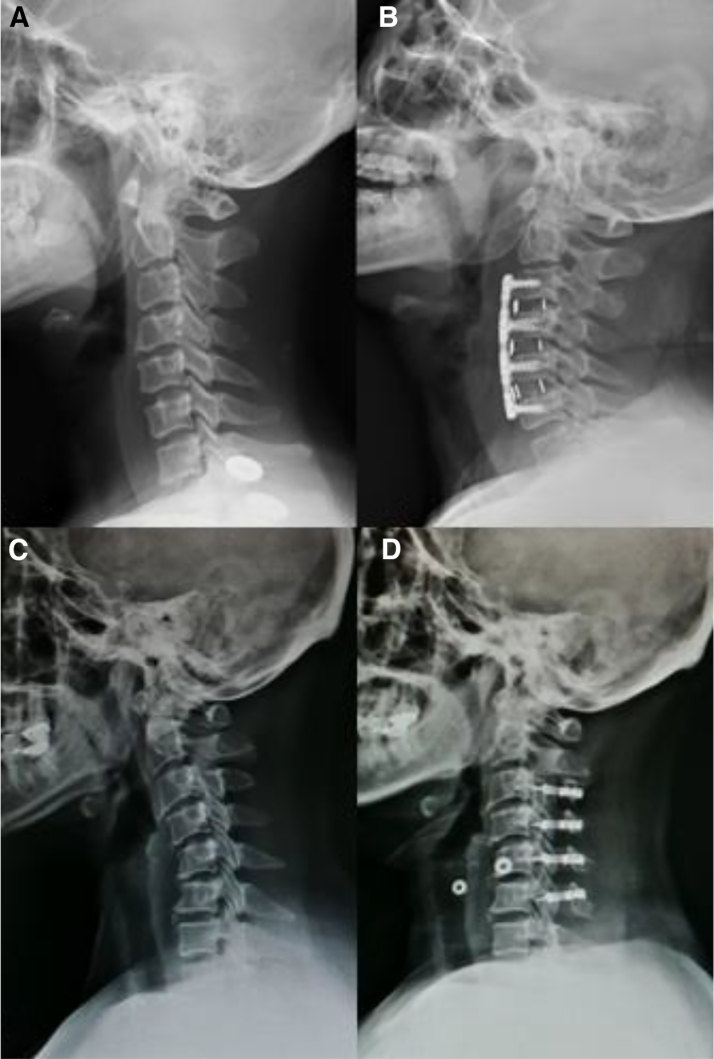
Multilevel anterior cervical discectomy and fusion (ACDF) and laminoplasty (LP) were performed to release the compression. Lateral x-rays of the cervical spine were taken in patients with multilevel cervical spondylotic myelopathy (MCSM) under ACDF preoperatively (A), at the 2-year follow-up visit (B), in patients with MCSM under LP preoperatively (C), and at the 2-year follow-up visit (D). ACDF = anterior cervical discectomy and fusion, LP = laminoplasty, MCSM = multilevel cervical spondylotic myelopathy.
